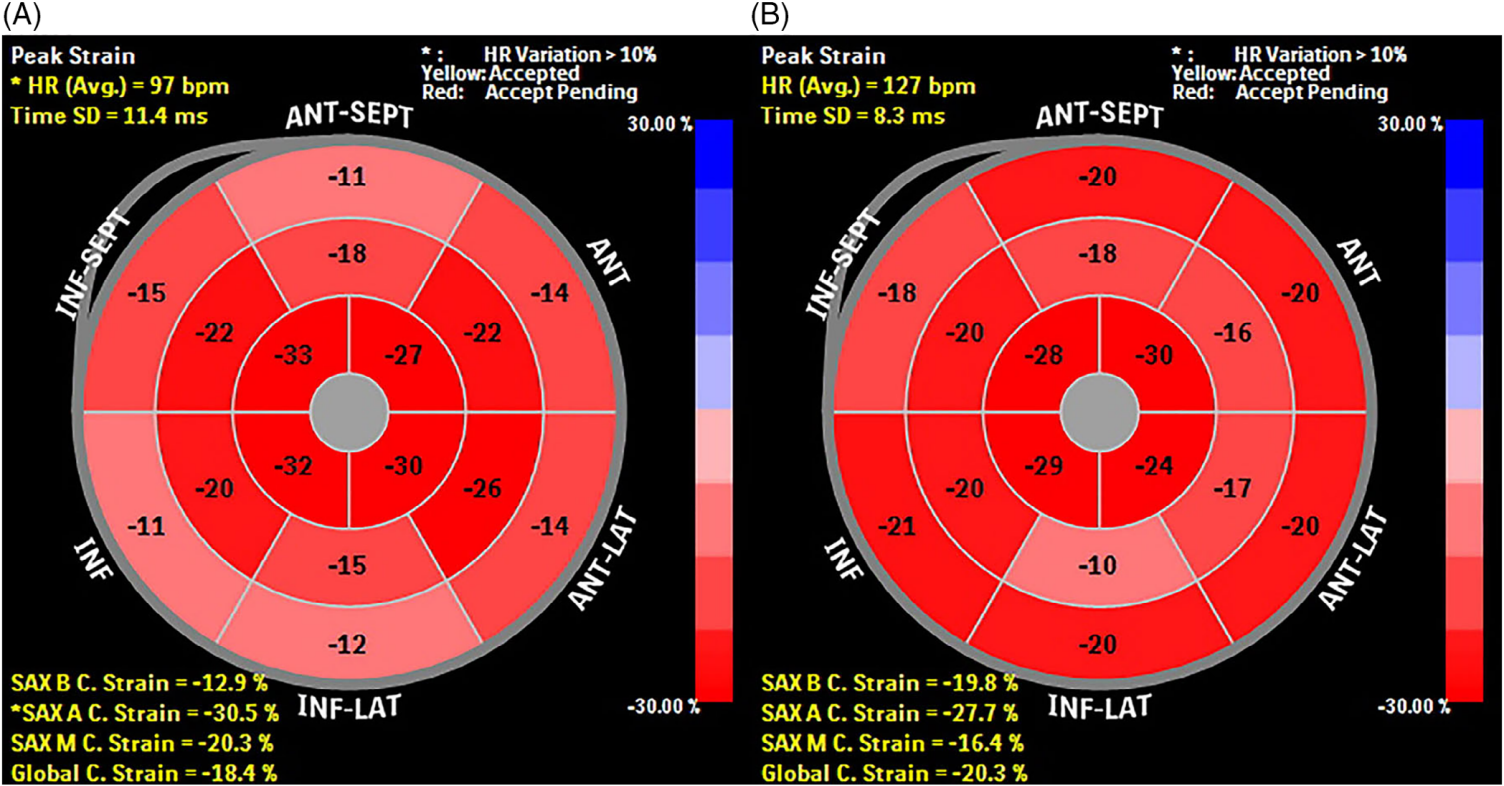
Representative examples of regional and global circumferential strain (GCS) derived from speckle tracking echocardiography (STE). A, Patient with resistant to intravenous immunology (IVIG). B, Patient with responsive to IVIG. The light red segments in A and B show the decrease in circumferential strain. HR, heart rate; Avg, average; SAX B C, short axis basal circumferential; SAX A C, short axis apical circumferential; SAX B C, short axis middle circumferential; C, circumferential
